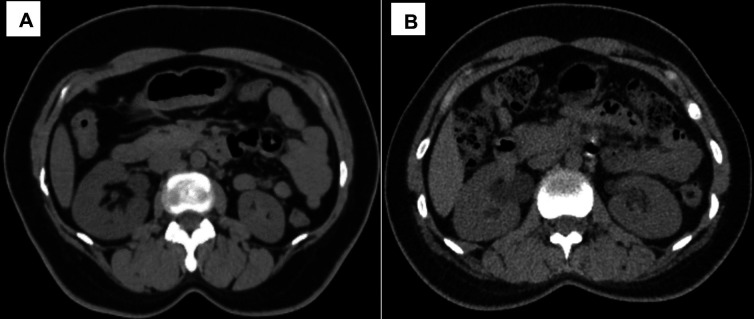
Axial CT KUB image acquired using AI-based automatic positioning technique (A). Axial CT KUB Image acquired using manual positioning technique (B).
